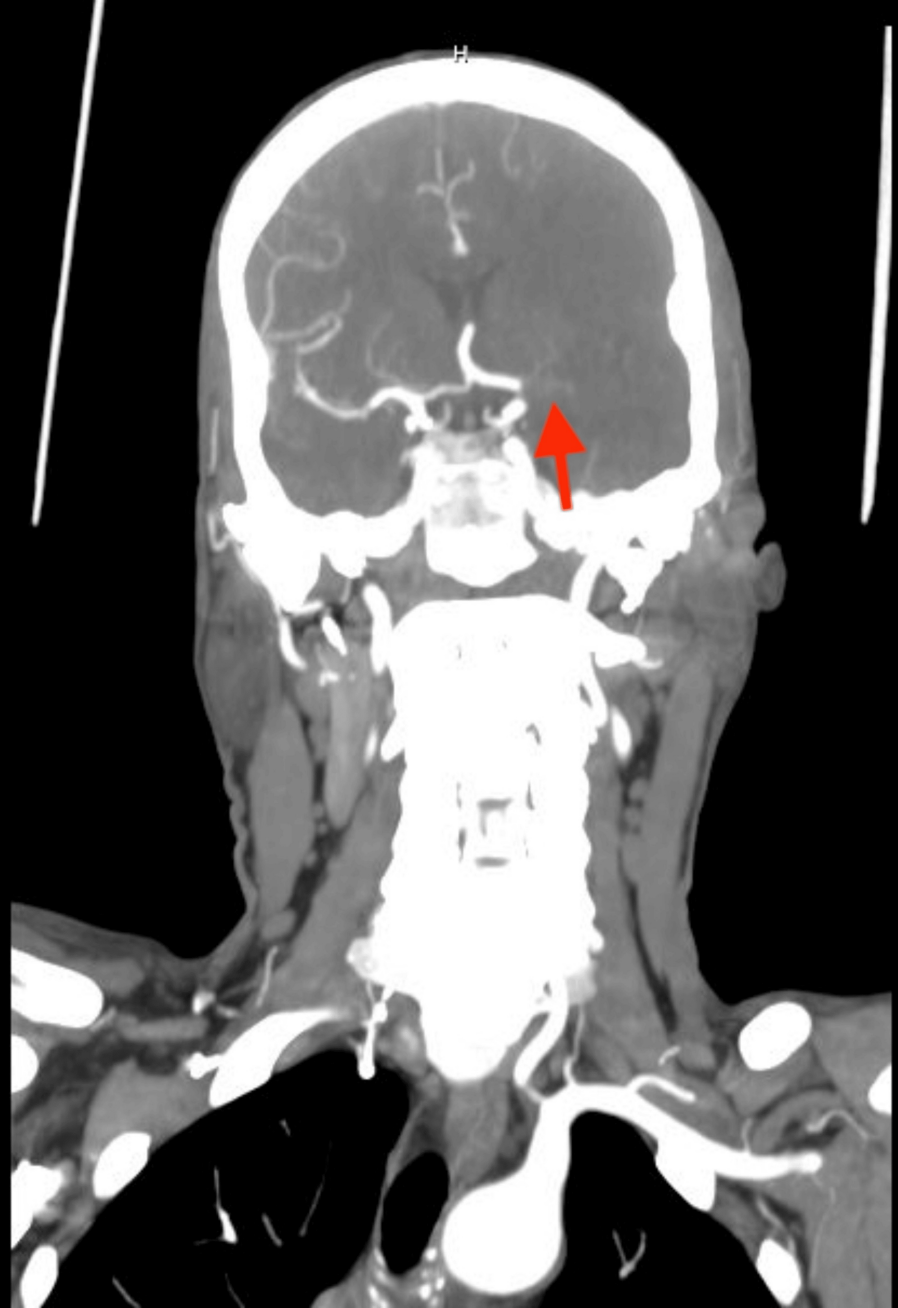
CT head angiogram shows no flow in the left middle cerebral artery (red arrow)
